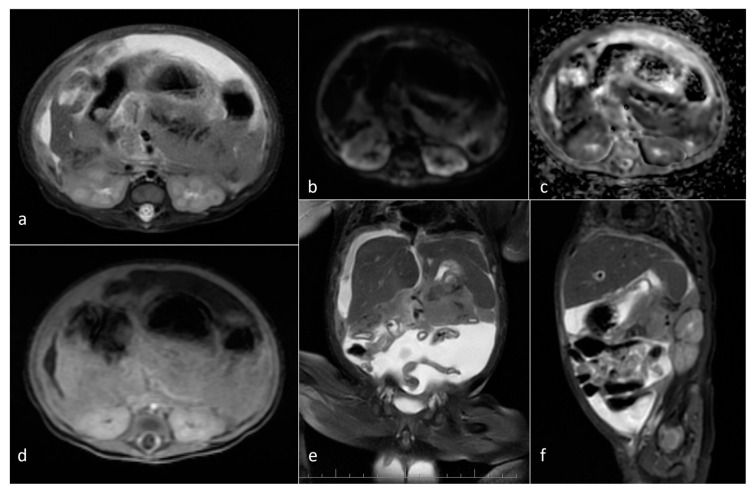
Juvenile xanthogranuloma. MRI of a neonate with irregular, solid, enhancing masses that are difficult to separate from internal organs. Increased volume of fluid in the abdominal cavity. (a,e,f) T2WI+fatsat: axial, coronal, and sagittal, respectively; (b) DWI; (c) corresponding ADC map; (d) axial CE-T1WI+fatsat.
